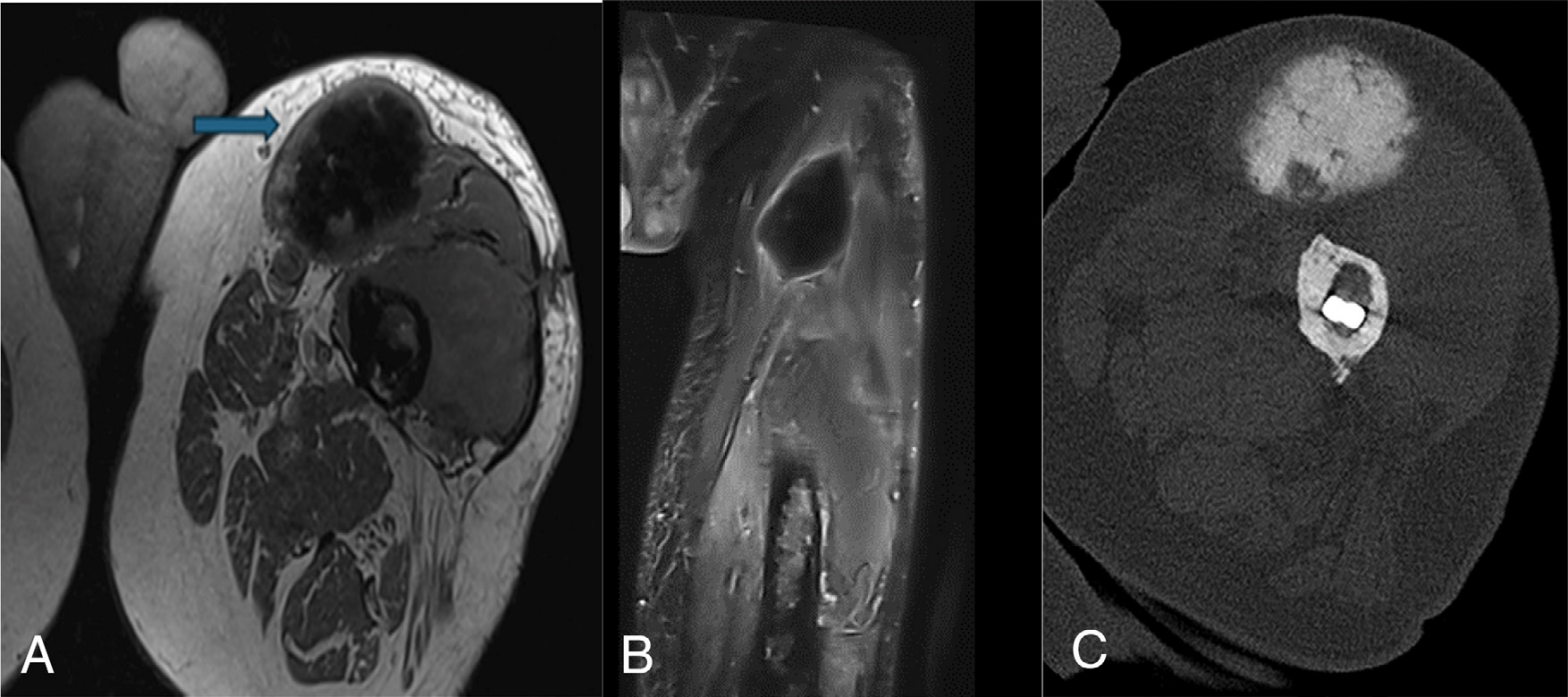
MRI. T2 DIXON (A) and post‑contrast T1 DIXON (B) sequences following intravenous gadolinium administration demonstrate a hypointense T1/T2 mass within the sartorius muscle with peripheral enhancement. (C) CT scan without injection in bone window. Individualization of an ossifying mass.
